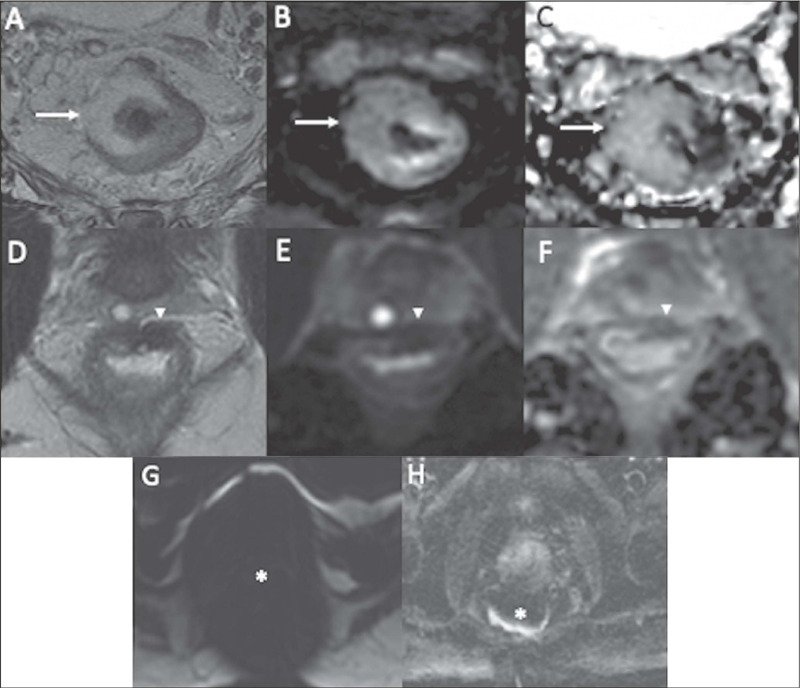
Examples of DWI artifacts. A-C: A T2 shine-through
